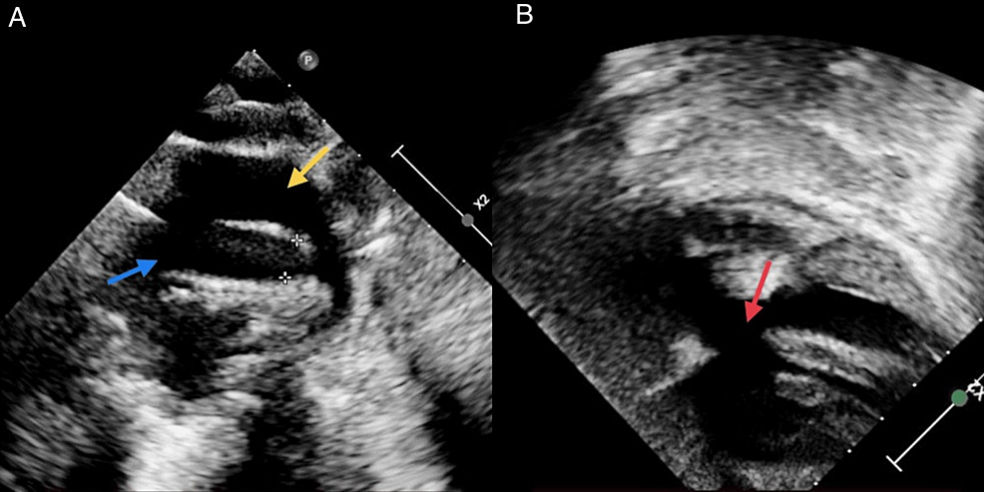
Echocardiography images showing (A) the left pulmonary artery (marked yellow) and ascending aorta (marked blue) and (B) showing VSD (marked red) The image was taken by the authors. VSD: ventricular septal defect
